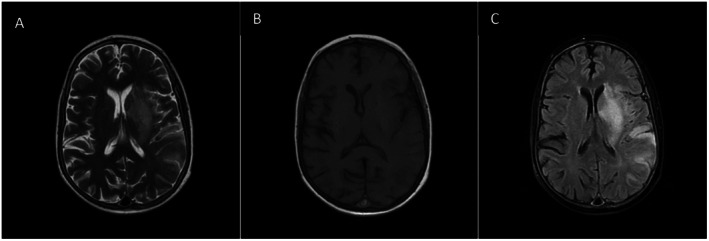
MRI Axial sequences (A–C). (A) Axial T2‐weighted image showing hyperintense signal in the left basal ganglia and insular cortex consistent with edema from acute infarction. (B) Axial T1‐weighted image demonstrating corresponding hypointensity in the same region. (C) Axial FLAIR sequence delineating the extent of the infarction involving the left corpus striatum, insula, and adjacent temporal–parietal cortex. Arrows indicate the affected area.
